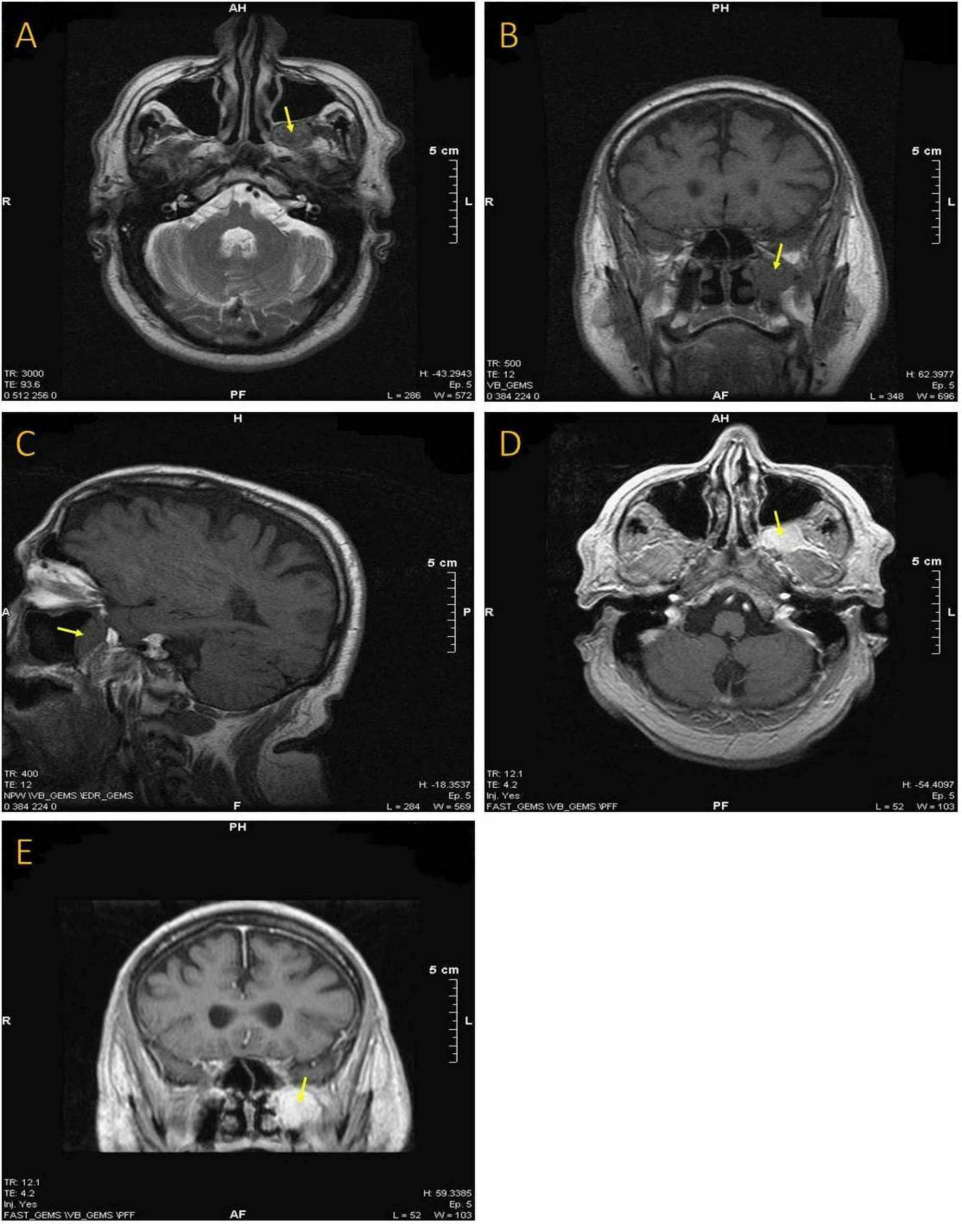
(A) An axial T2-weighted with fat suppression MRI scan showing the lesion located in the left pterygopalatine fossa. (B) A coronal T1-weighted MRI showing the sizeable mass in the same patient. (C) A sagittal T1-weighted MRI demonstrating the lesion located just behind the posterior wall of the left maxillary sinus. (D) and (E) Axial and coronal T1-weighted MRI with gadolinium.
